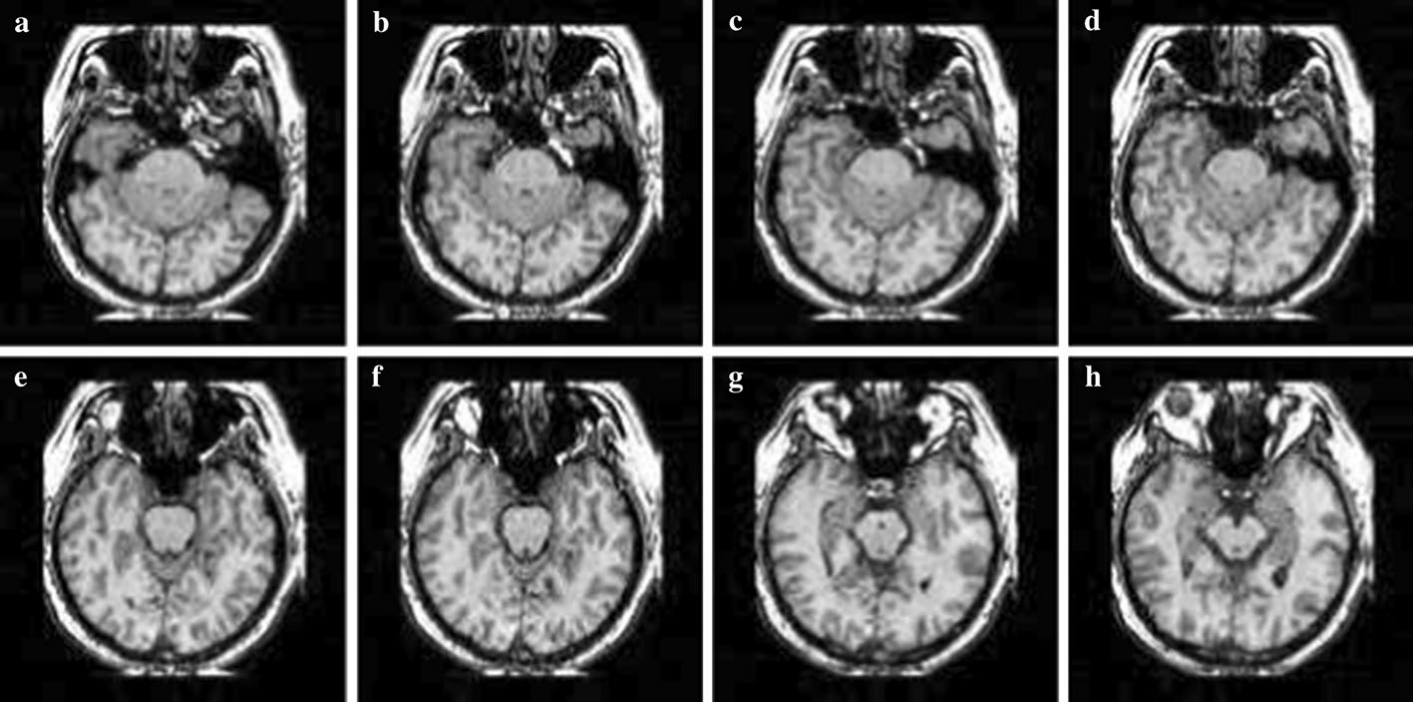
Compressed MRI image slices (a–h) by 3D-HLCK at a BR = 1.0 bpp
